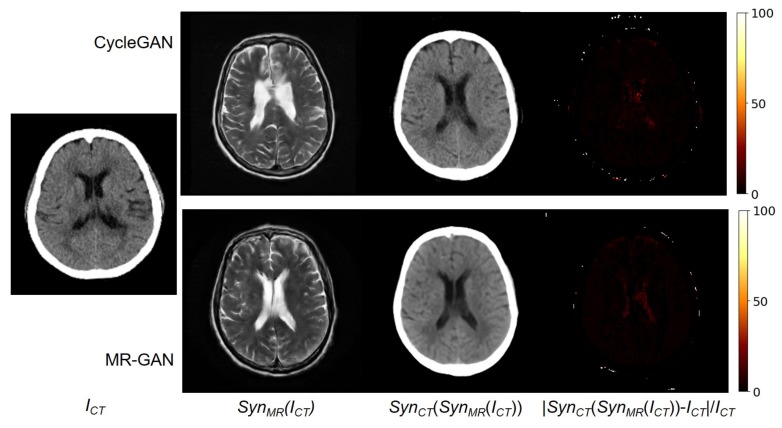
From (left) to (right): Input CT image, synthesized MR image, reconstructed CT image, and relative difference error between the input and reconstructed CT images.
